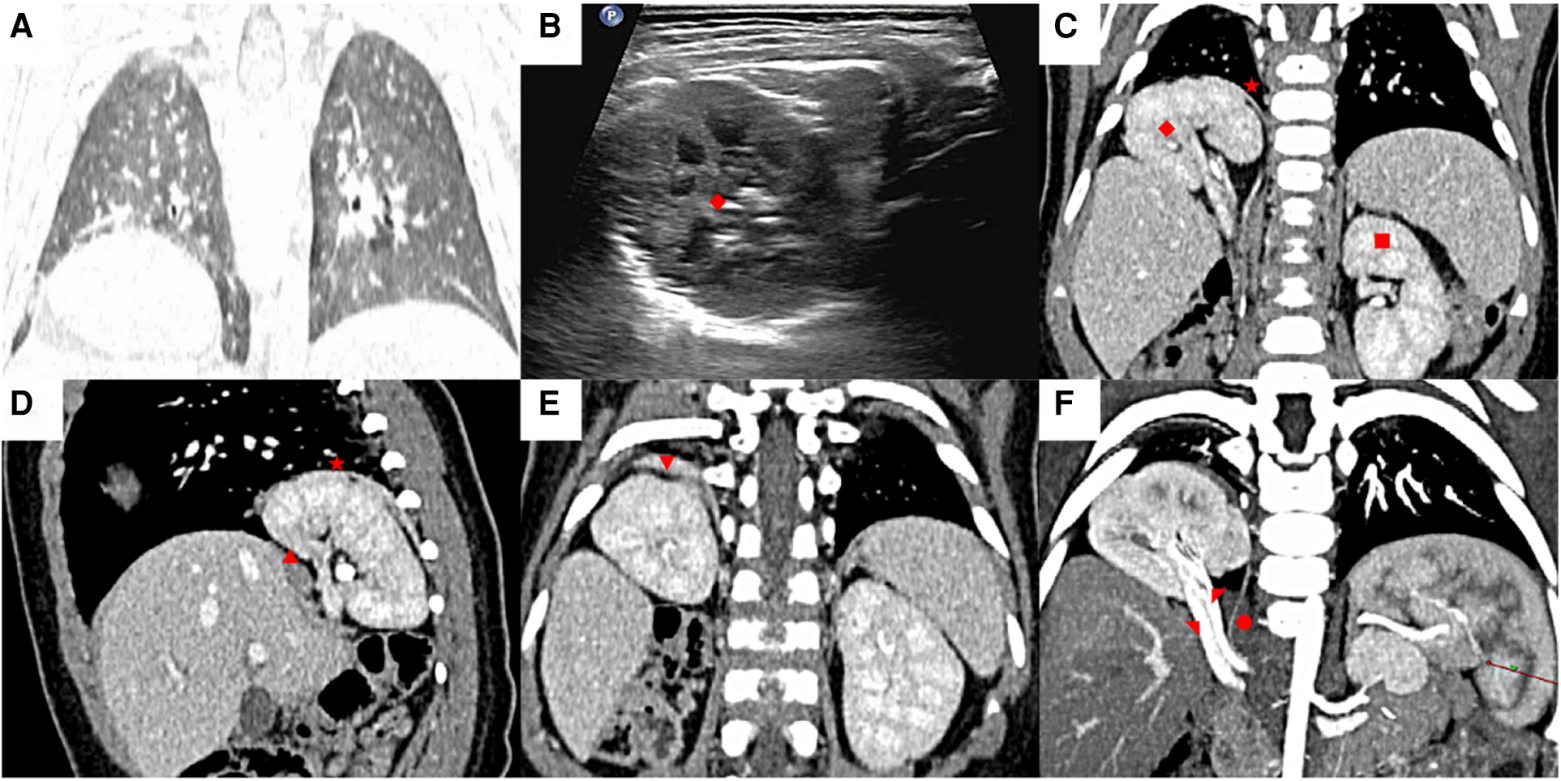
Preoperative ultrasound and chest computed tomography (CT) imaging findings. (A) The chest CT coronal image showed right diaphragmatic elevation, suspected an abnormal mass herniated into the chest cavity. (B) Chest ultrasound confirmed that the right kidney had herniated into the right chest cavity. (C) Contrast enhanced chest CT scan showed that the rotation position of the right kidney was significantly shifted upwards above the diaphragm (coronal position). (D) The local discontinuity in the right diaphragm (sagittal position). (E) Thickening of the upper wall of the hernia sac with significant enhancement on enhanced scanning. (F) The abnormal supply of small blood vessel originating from the right renal artery. (◆: Right kidney, ★: Hernia sac, ▪: Left kidney, ▴: Diaphragmatic muscle, ▾: Infradiaphragmatic pulmonary sequestration, ●: Abnormal blood vessels, ◤: Right kidney artery, ◥: Right kidney vein).
